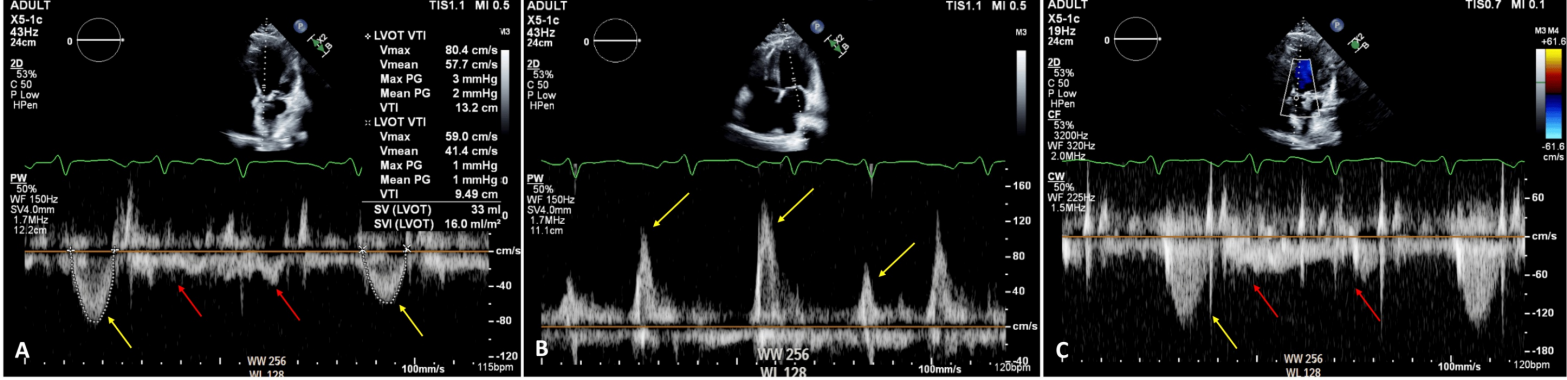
Echocardiographic findings. A: Left ventricular (LV) outflow tract pulsed wave Doppler showing beat-to-beat variation in systolic flow (yellow arrow). Notice significantly reduced absent flow in multiple consecutive beats (red arrow). B: Pulsed wave Doppler through mitral valve demonstrating diastolic beat-to-beat variation in LV inflow (yellow arrow). C: Continuous wave Doppler through the aortic valve demonstrating Doppler alternans (yellow arrow) and significantly reduced systolic flow on multiple beats (red arrow).
